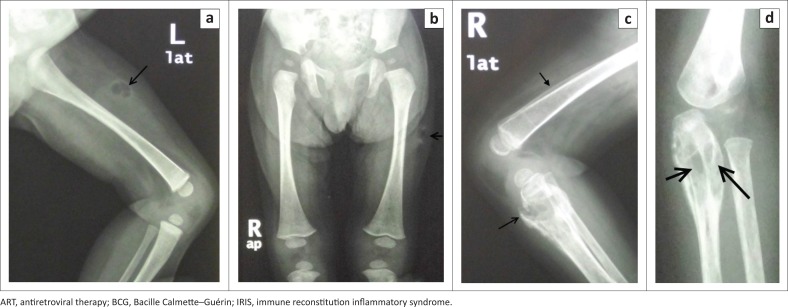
A 9-month-old HIV-infected girl, with CD4 count 9 cells/mm^3^ (1.53%), was admitted with severe malnutrition, right axillary and left thigh abscesses, generalised lymphadenopathy, hepatosplenomegaly and clinical evidence of HIV encephalopathy. ART was initiated with subsequent worsening of the thigh abscess. An aspiration was performed on the left thigh abscess which cultured *Mycobacterium bovis* BCG, confirmed using molecular testing. Appropriate antimycobacterial treatment was provided: (a) (lateral) and (b) (AP), radiograph of the lower limbs demonstrate an air containing abscess in the upper left lateral thigh soft tissues (open arrow) and generalised left lower limb soft tissue swelling. No periosteal reaction was demonstrated in visualised bones. She was readmitted a year later, still on antimycobacterial treatment with soft tissue abscesses in the right thigh, forehead, abdominal wall and left forearm; (c) (left lateral knee) demonstrates osteomyelitis, with lytic lesions within the proximal tibial metaphysis and a cortical break along the anterosuperior tibial margin (open arrow). There is periosteal reaction along the tibia and distal femur (closed arrow) with generalised soft tissue oedema; (d) (left AP forearm) demonstrates another focus of osteomyelitis with lytic lesions in the proximal metadiaphysis of the ulna (open arrows), associated periosteal reaction and osteopenia of the left ulna and radius. Bone aspiration again confirmed *M. bovis* BCG, now resistant to first-line antimycobacterial agents, necessitating surgical drainage; however, the patient did not survive. A diagnosis of BCG-IRIS was made.
